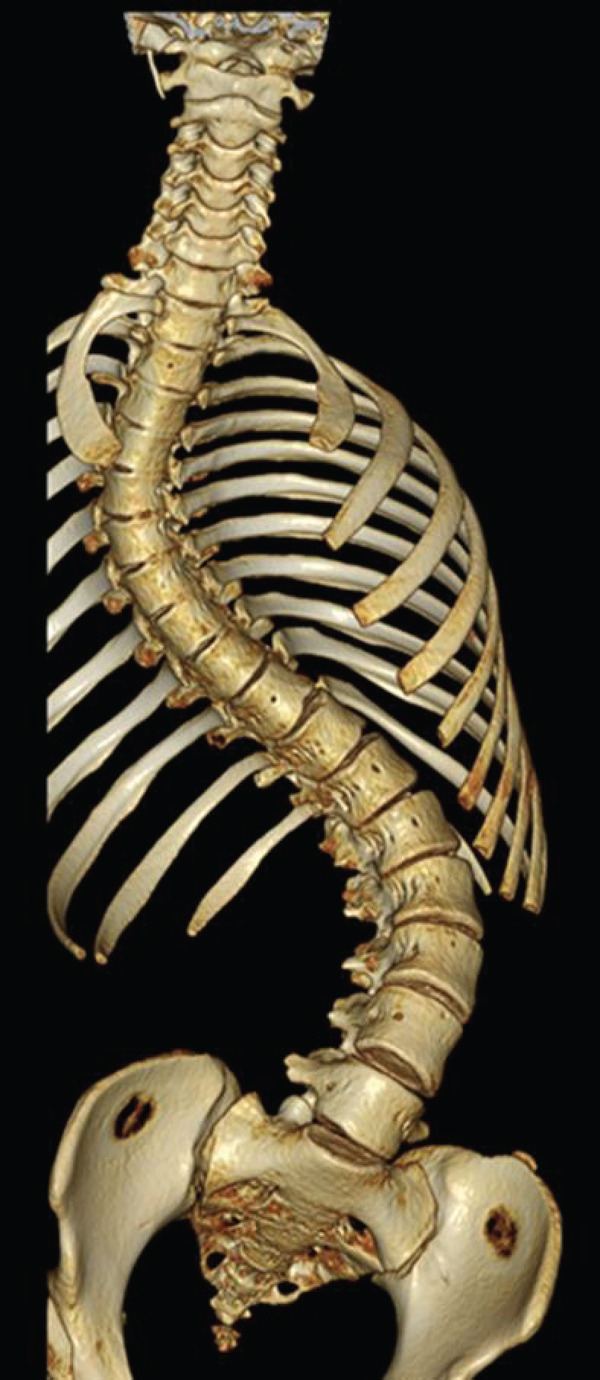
Preoperative three‐dimensional computed tomography of the full spine.
